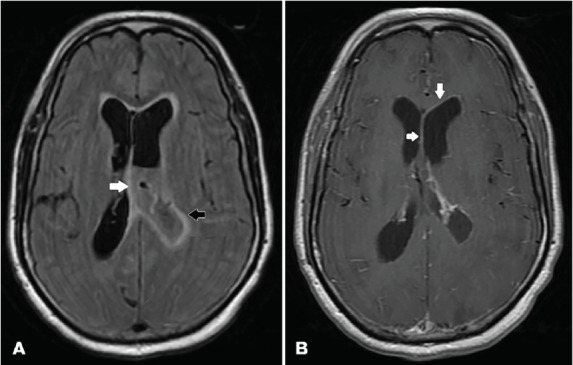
A) Axial magnetic resonance imaging (MRI) (fluid-attenuated inversion recovery sequence) showing mild enlargement of the left lateral ventricle, with debris and purulence in the ventricle (white arrow). Mild edema and transependymal flow of cerebrospinal fluid surrounding both ventricles are present (black arrow). B) Axial MRI without contrast showing enhancement of the left ventricle (white arrows).
